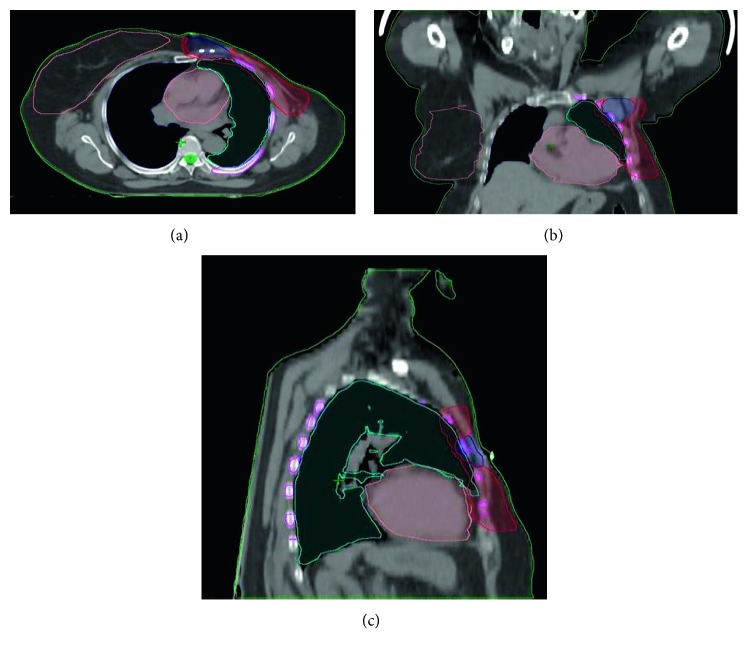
Axial, coronal, and sagittal planes of view from the CT data set of the patient, including target and OAR structures.
